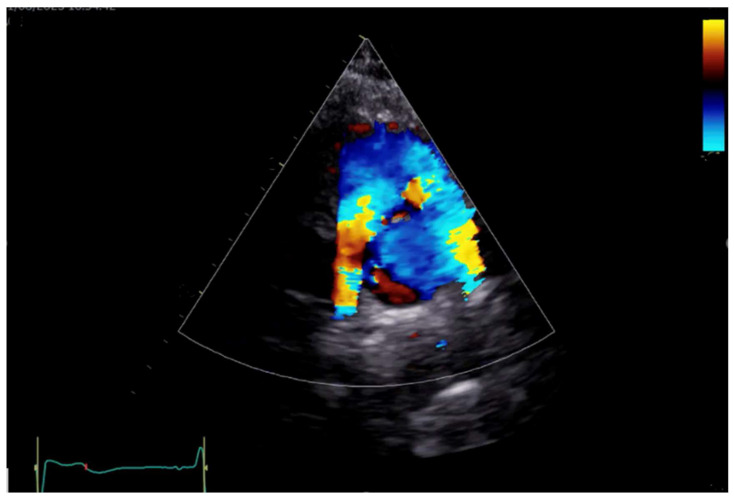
High-parasternal view showing color Doppler at level of pulmonary branches after LeCompte maneuver.
